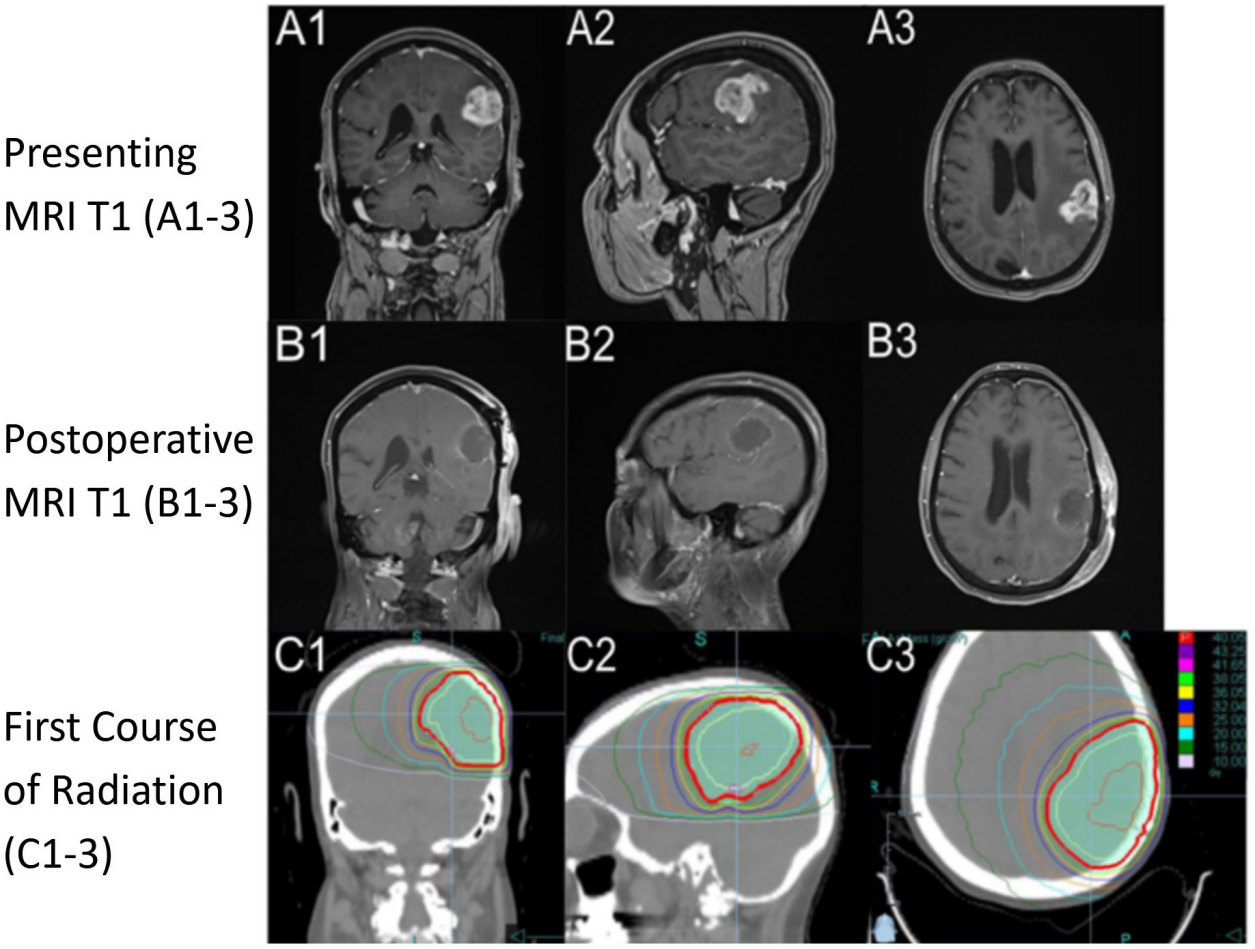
Imaging from presentation with T1 sequence on (A1-A3) demonstrating a hyperintense ring-enhancing lesion in the left frontal-parietal region. Postoperative imaging, day 7 after diagnosis, with T1 sequence on (B1-B3) demonstrating postoperative changes. RT plan 1 (RT1) utilizing a noncontrast computed tomography (CT) with dose color outline. First course of RT (C1-C3) (red is 40.05 Gy, purple is 43.25 Gy, pink 41.65 Gy, light green 38.05 Gy, yellow is 36.05 Gy, blue is 32.04 Gy, orange is 25.00 Gy, light blue is 20.00 Gy, dark green is 15.00 Gy, and light pink is 10.00 Gy). Column 1 is a coronal section through the body of caudate and anterior cerebellum, column 2 is a sagittal section through the left superficial temporal and parietal lobe, and column 3 is an axial section through superior cerebrum and lateral ventricles.
